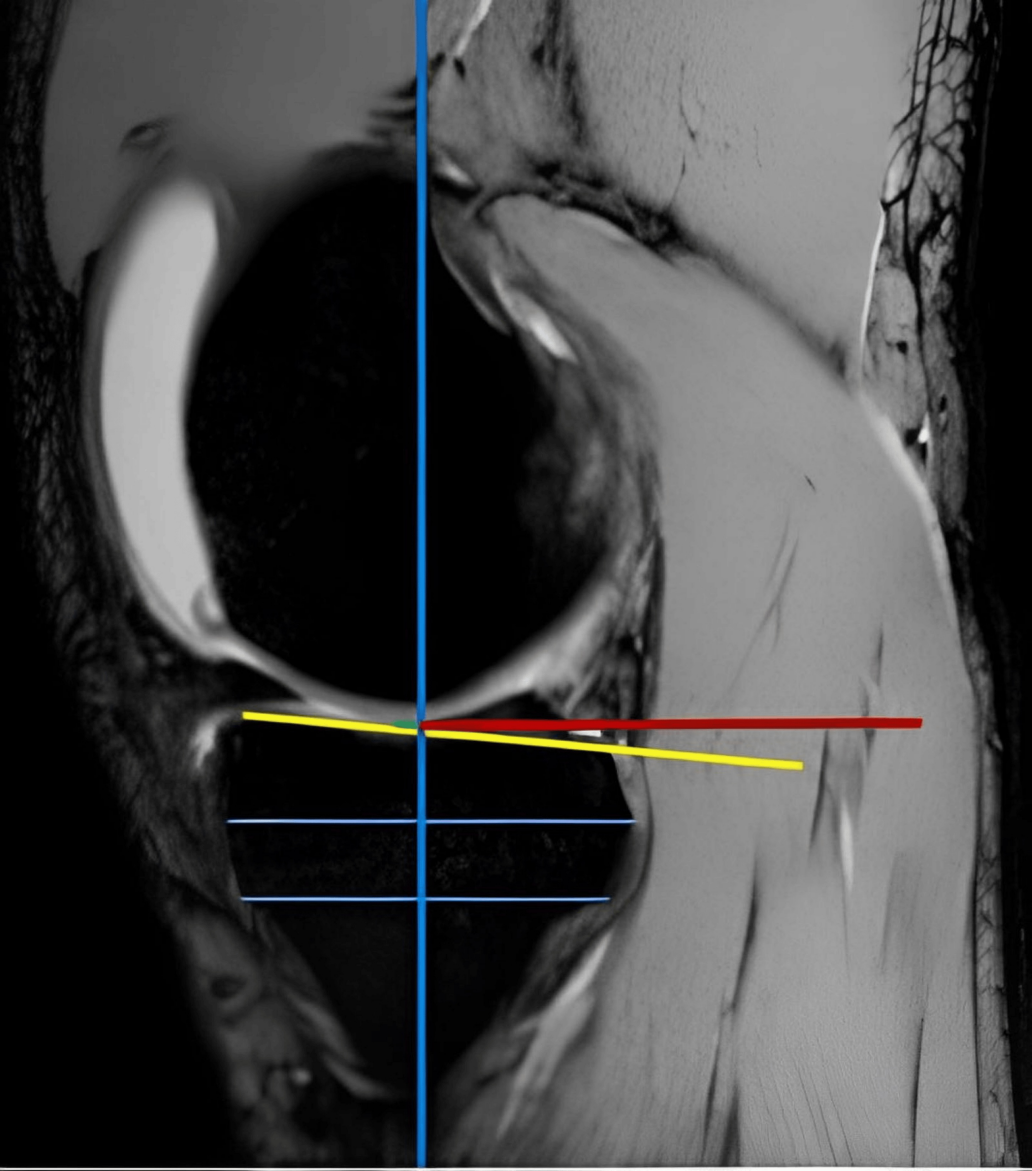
Tibial slope measurement in MRI scans.
